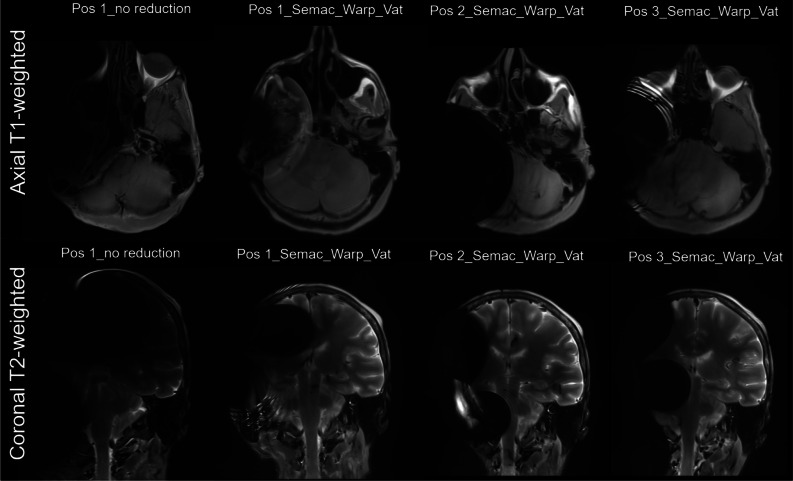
Representative visualisation of artefacts in axial plane/T1-weighted (top row) and coronal plane/T2-weighted (bottom row) without and with SEMAC-VAT WARP Metal Artefact Reduction Sequences (mars) for the 3 different implant placements. Headers indicate the implant position (Pos 1–3) and the measurement with no reduction or with MARS.
